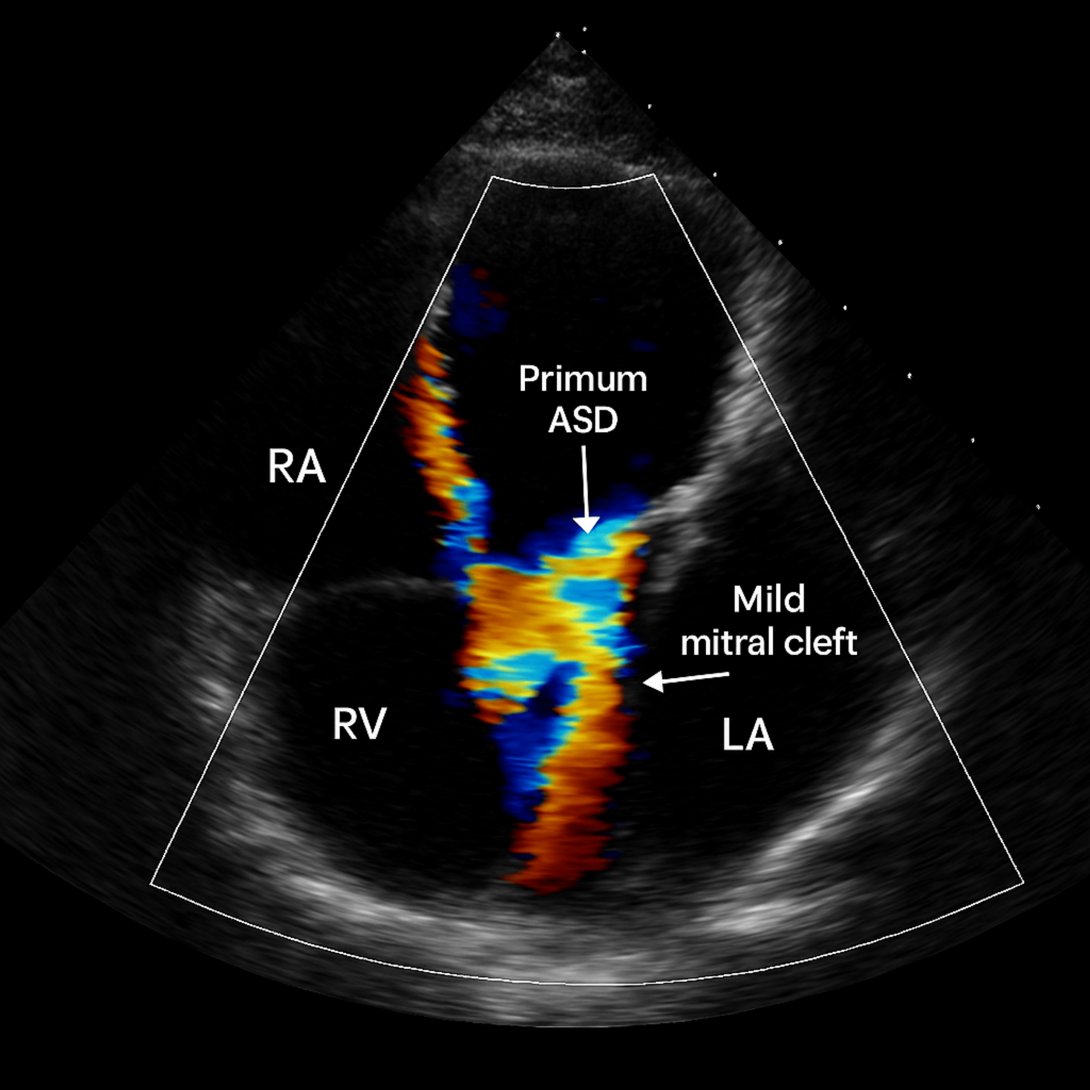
Bubble contrast echocardiography revealed early opacification of the left atrium, mild mitral cleft and primum ASD RA: right atrium, RV: right ventricle, LA: left atrium, ASD: atrial septal defect
